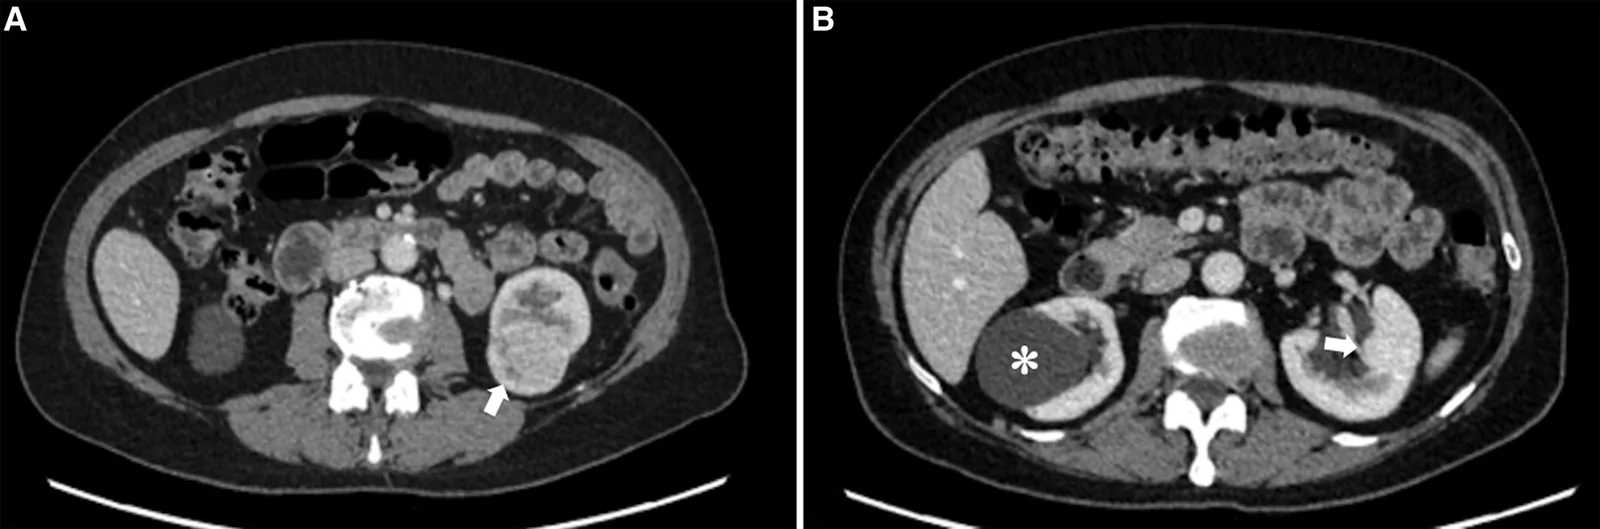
Axial contrast-enhanced CT images demonstrating a dorsally located mass measuring up to 48 mm in the lower pole of the left kidney (*A*, arrow), visualized again in a more cranial slice (*B*, arrow), together with a right renal parenchymal cyst measuring up to 57 mm (*B*, asterisk).
